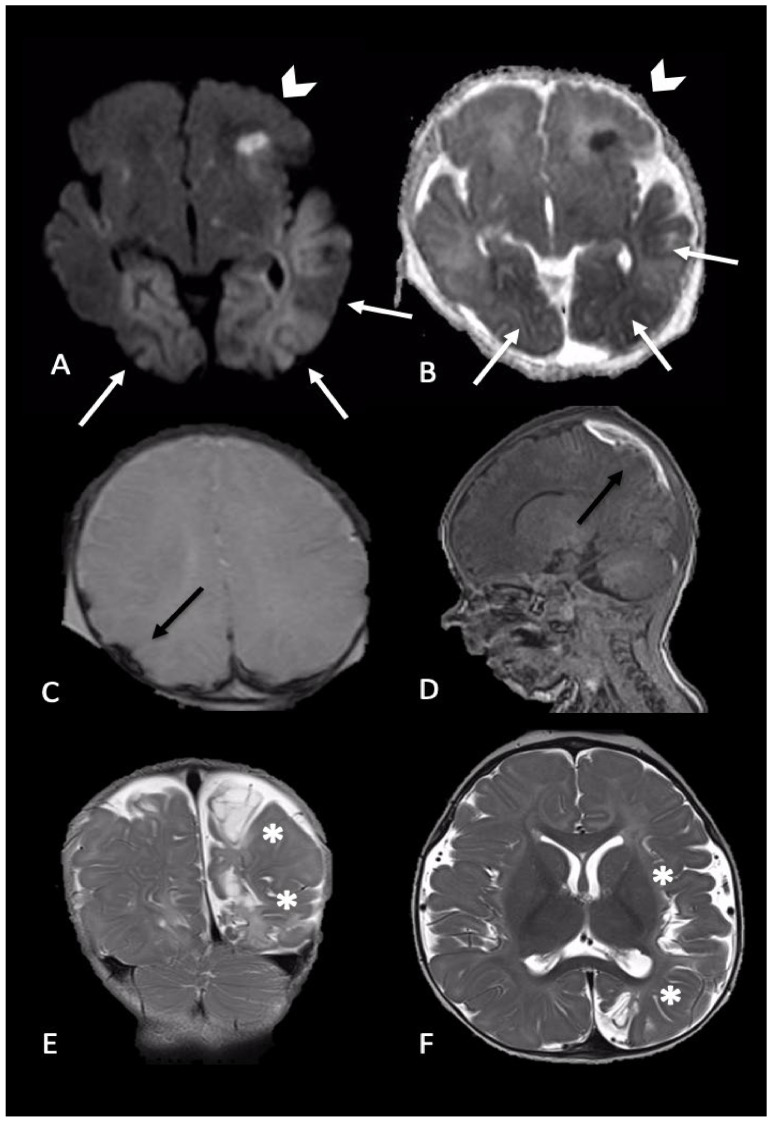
Newborn reached the emergency department for single episode of apnea followed by generalized seizures. MRI performed 4 days later showed intraparenchymal injuries and bilateral extra-axial mixed-intensity fluid collections. In particular, (A,B) (axial DWI and ADC maps) showed bilateral temporal and occipital cortical areas of restricted diffusion (white arrows) and a focal area of restricted diffusion localized in the left frontal lobe and diffuse (arrows-head), indicative of acute intraparenchymal injury; (C,D) (axial SWI and sagittal T1-W) showed extra-axial blood collection along the parietal convexity bilaterally (black arrows). Six-month follow-up MRI ((E,F), coronal and axial T2-W) revealed malacic evolution of intraparenchymal damage and consequent expansion of extra-axial spaces due to tissue loss (asterisks).
